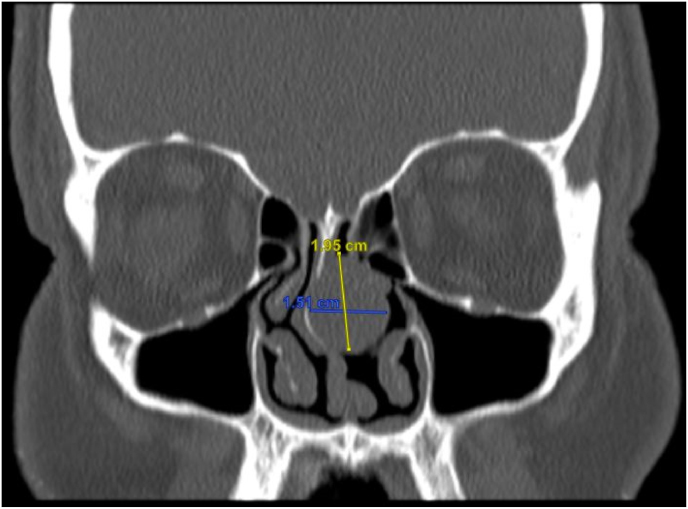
Computed tomography scan (coronal view) of the paranasal sinuses showing a well-defined soft tissue density lesion in the left nasal cavity, measuring 1.95 × 1.51 cm.
